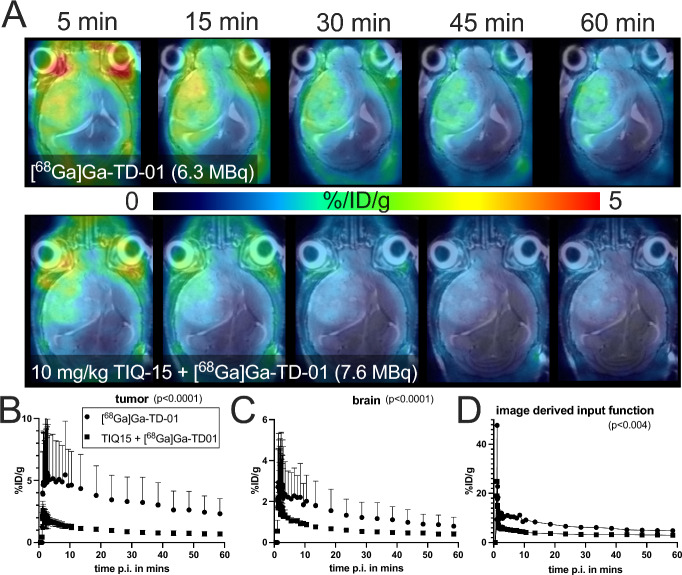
(A) Representative PET/MRI time series of baseline (upper panel) or receptor blocking study (lower panel) following i.v. injection of [^68^Ga]Ga-TD-01 only or by pre-blocking with TIQ15 (10 mg/kg). (B) Tumor TAC for baseline and blocking condition showing significant (t-test, *p* < 0.0001) lower uptake of [^68^Ga]Ga-TD-01in tumor following pre-blocking with TIQ15. C) Healthy brain TAC following baseline and blocking conditions with significant lower uptake of [^68^Ga]Ga-TD-01 after receptor pre-blocking with TIQ15 (t-test, *p* < 0.0001). D) Input function (mean) based on time activity data derived from vena cava. (B–D) Data are expressed as %ID/g and presented as mean ± SD (n = 13)
